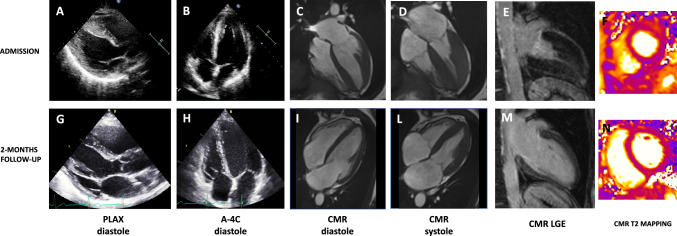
Echocardiographic and cardiac magnetic resonance presentation and evolution at two month. On Panel A and B the echocardiographic parasternal long axis and apical 4 chamber views at the admission are presented. In panel C, D, E and F CMR results are shown, particularly diastolic apical 4 chamber view (C), systolic apical 4 chamber view (D), 2 chamber LGE contrast imaging (E) and T2 contrast mapping at the medioventricular level showing high relaxation times (F). On the bottom, evolution at 2 months is represented. In panel G and H, the follow-up the parasternal long axis and apical 4 chamber views are presented, showing regression of the ‘pseudo-apical hypertrophy’. In panel I, L, M, N CMR results are shown, particularly diastolic apical 4 chamber view (L), systolic apical 4 chamber view (M), the 2-chamber LGE contrast imaging and T2 contrast mapping at the medio-ventricular to basal level with normalized T2 values (N)
